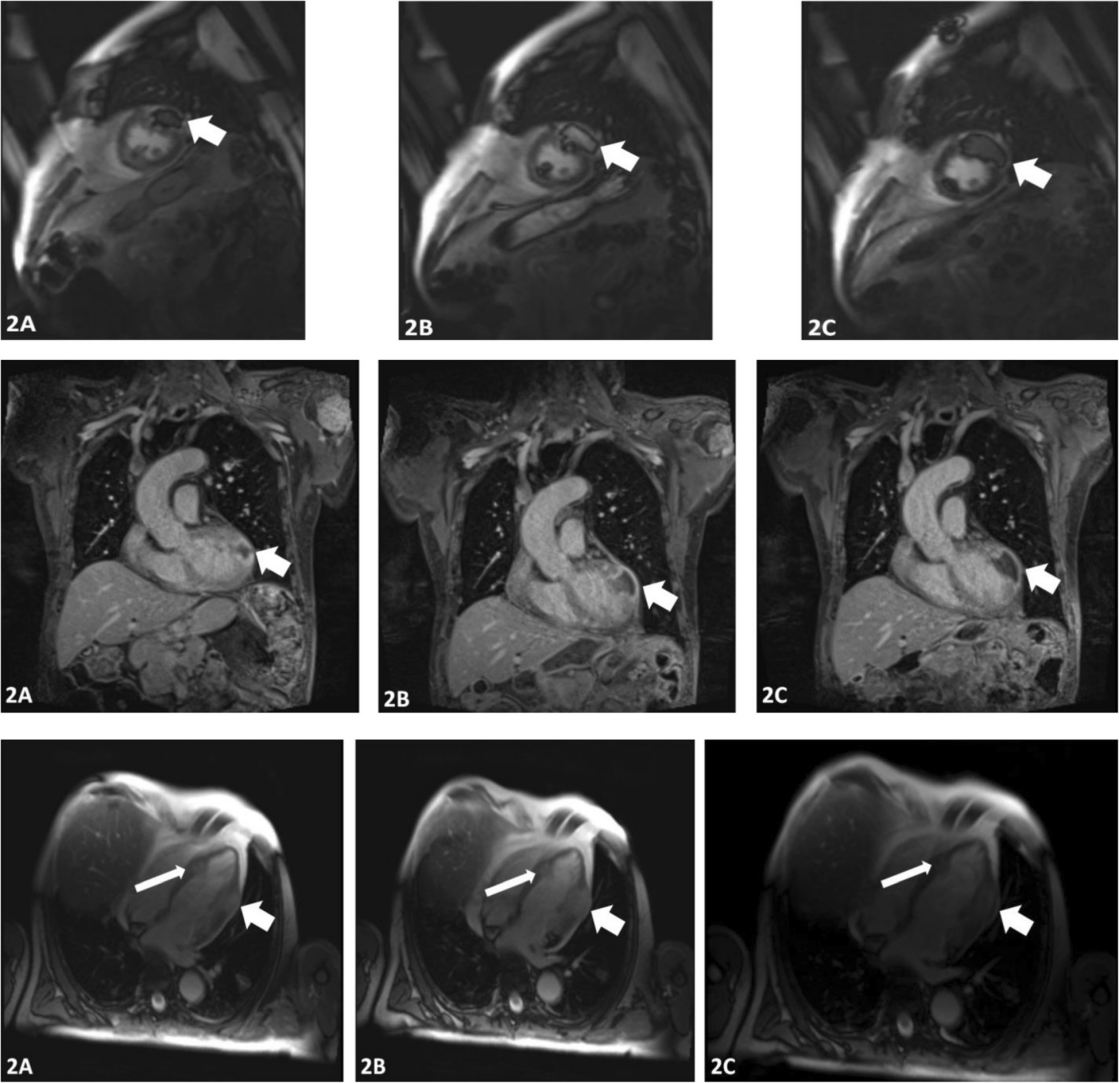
Cardiac-MRI performed on treatment onset and follow-up showing multiple (2A, 2B, and 2C) intramyocardial tumors and large necrosis on TKI treatment - coronal, horizontal and sagittal sections.
